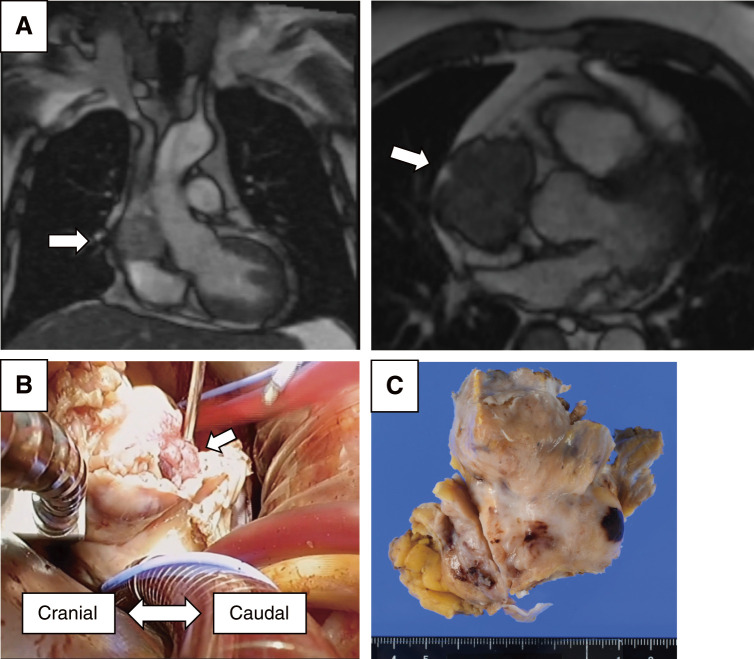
Surgical finding of metastatic adenocarcinoma. (Patient No. 15) Preoperative contrast-enhanced magnetic resonance imaging showed a large mass occupying the right atrium (panel A, arrows). Intraoperative findings are shown in panel B. Through a right fourth intercostal thoracotomy, the tumor extensively and firmly infiltrated the right atrial wall, extending to the roof of the left atrium. Cardiopulmonary bypass was established via the right femoral artery and vein. The right atrial wall was resected en bloc. A firm, white tumor measuring 55 × 38 mm was completely excised (panel C).
